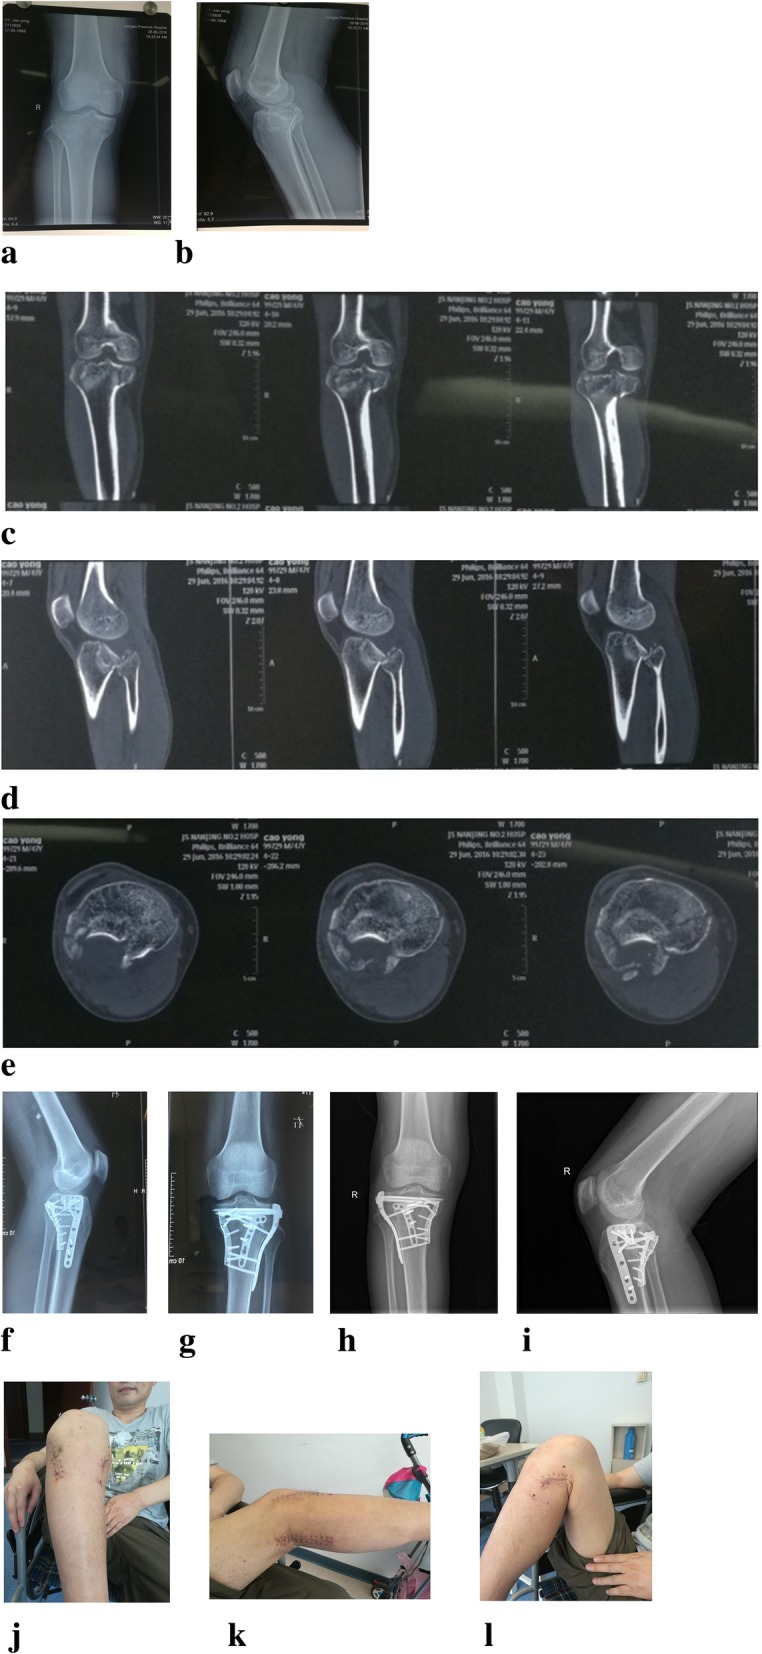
a–c A typical case of the three-incision combined approach for complex fracture of tibial plateau involving the posterolateral tibial plateau. A male 41 case suffered traffic accidents, and preoperative X-ray indicated tibial plateau fracture, which was of type V according to Schatzker classification. Further CT examination indicated the fracture affected all the three columns, and three-incision combined approach for fracture reduction and fixation was performed. Follow-up results at 1 year after surgery showed good recovery of the patient, with good flexion and extension of the knee joint. a-b: pre-op X-ray; c-e: pre-op CT; f-g: post-op X-ray immediate; h-i: post-op X-ray after one year follow-up; j-l: Functional posture image one year follow-up after operation
